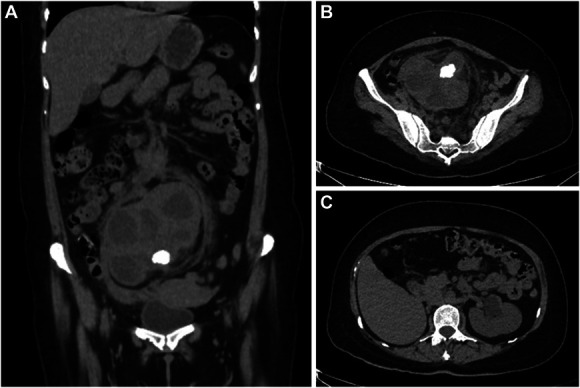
CT Urogram images. (A) Coronal plane of the CT urogram. The ectopic kidney is seen in the lower abdomen. It is enlarged because of hydronephrosis, caused by the obstructing renal stone seen at the hilum. (B) Transverse plane of CT urogram (lower abdomen). The hydronephrotic ectopic kidney is again seen, together with the kidney stone in its pelvis and perirenal fatty striation. (C) Transverse plane of the CT urogram. The left kidney is located in a normal position. The presence of mild–moderate hydronephrosis with mild ureteral ectasia is also evident. CT, computerized tomography.
